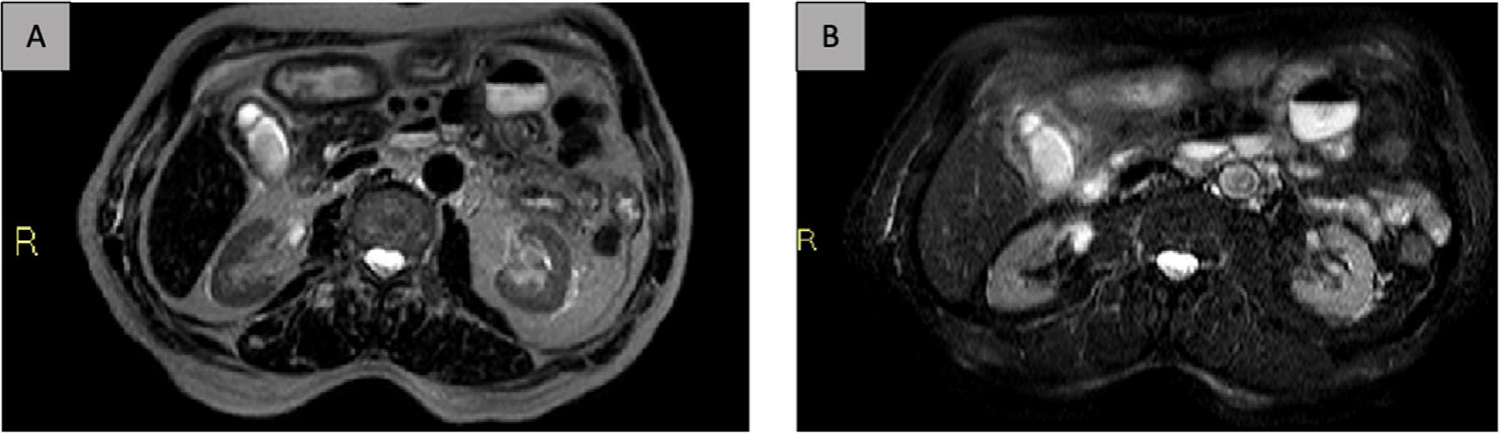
Axial MR image of the abdomen (A. T2w, B. SPAIR) demonstrated fluid collection in the gallbladder bed in a chronic inflammation background it.
